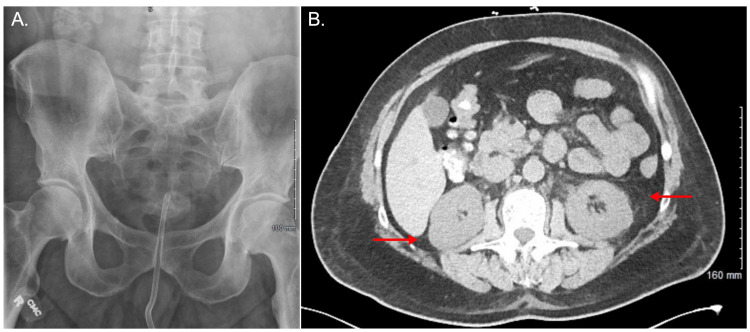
KUB and abdominal CT on presentation to the emergency department (A) KUB confirming catheter placement with no significant findings. (B) CT showing mild perinephric fat stranding bilaterally (red arrows) without signs of urinary obstruction. KUB: kidney, ureter, and bladder radiography
